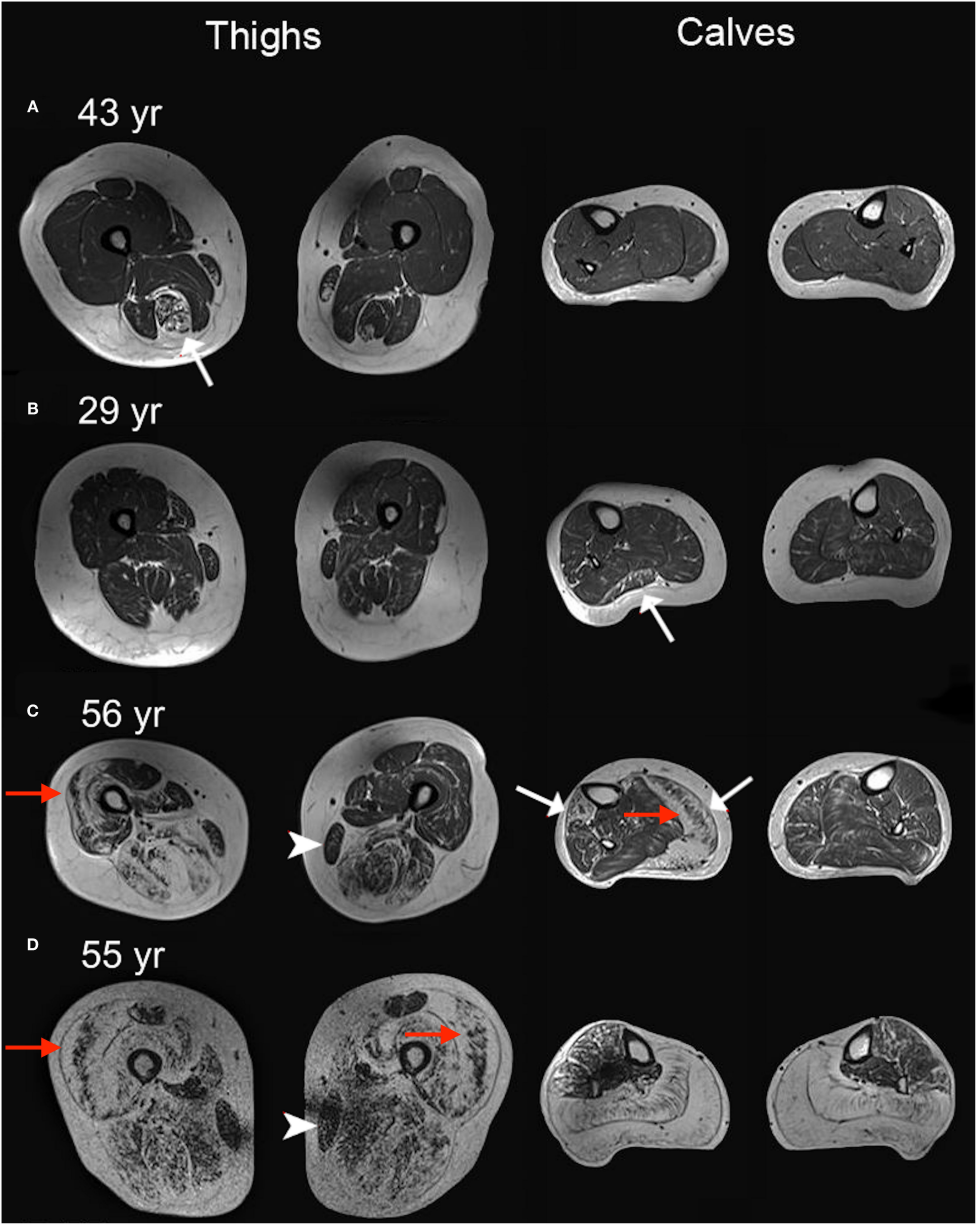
Four T1-weighted examples. T1-weighted MRI images of thighs and calves of four women heterozygote for pathogenic *DMD* variants. White represents fat tissue. (A–D) shows a range of muscle affection from mild to severe. Cases (C,D) are particularly affected with prominent replacement of muscle by fat. Correspondingly, muscle strength was compromised in these patients. Cases (A–C) have asymmetric fatty replacement (white arrows) in one or more muscles. Note the sparing of the gracilis muscle in cases (C,D) (arrowheads). Sandwich sign (preserved core of muscle surrounded by fat replaced muscle), reminiscent of what is seen in Bethlem myopathy, is observed in several muscles (red arrows). For more details on these four cases, see Table 1: subject no. 3 for (A); subject no. 34 for (B); subject no. 23 for (C); subject no. 32 for (D).
